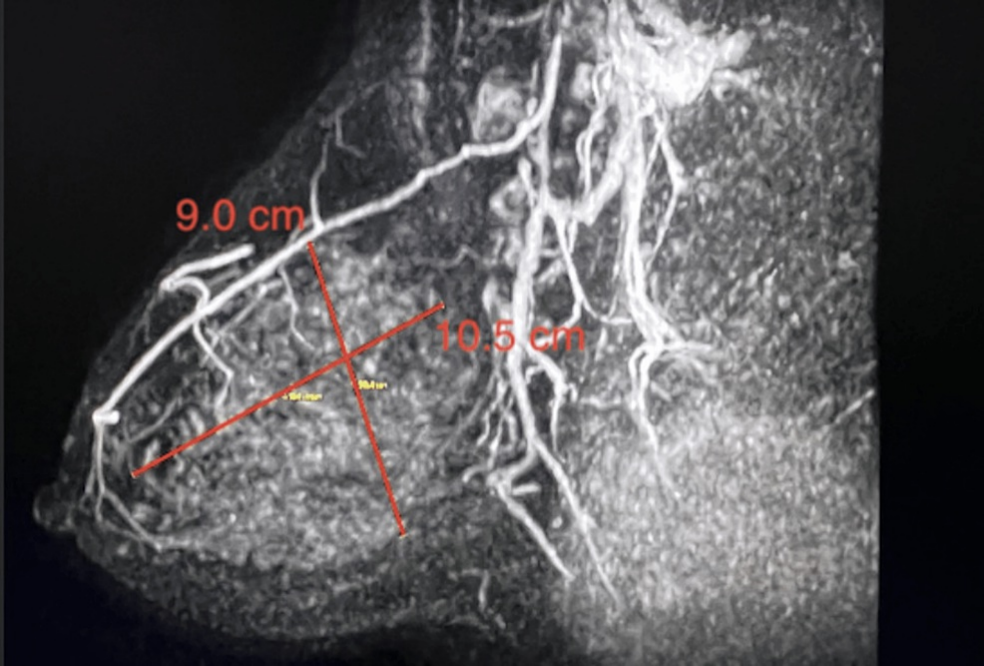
Lateral MRI of the breast showing a 9x10.5x4.6 cm non-mass-like enhancement in the right breast
